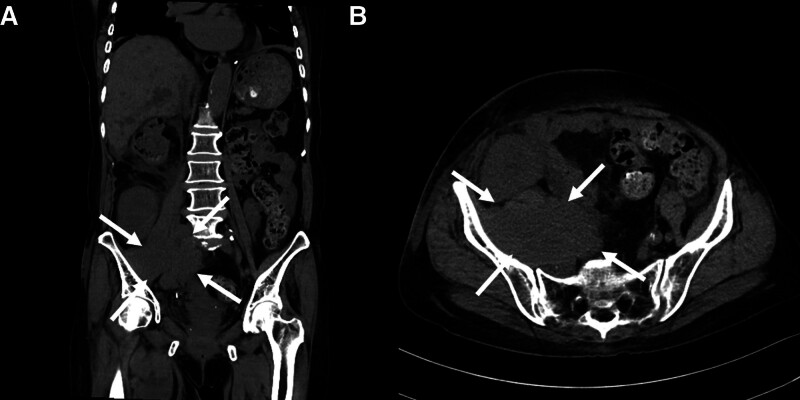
Contrast-enhanced CT scan revealing retroperitoneal mass compressing the iliac vessels ((A) coronal view and (B) axial view).
